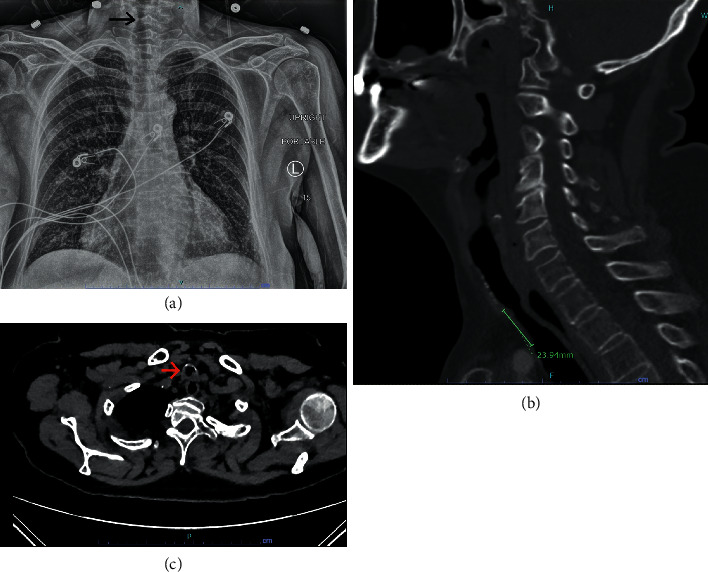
(a) Chest radiograph showing the stenosis in the upper third of the trachea; (b) sagittal section of the trachea showing the length of the stenotic segment; (c) axial cut of the narrowest segment of the trachea.
